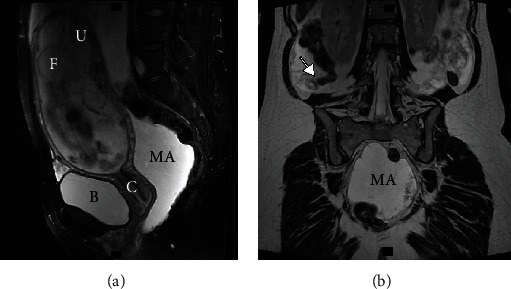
(a) Abdominal and pelvic MRI (sagittal plane) without IV contrast shows a gravid uterus and a substantial amount of T2 hyperintense fluid. (b) Abdominal and pelvic MRI (coronal plane) without IV contrast demonstrates an enlarged perforated appendix with extraluminal mucin (arrow) and T2 hyperintense fluid collection in the pelvis. B: bladder; C: cervix; F: fetus; IV: intravenous; MA: mucinous ascites; MRI: magnetic resonance imaging; U: uterus.
